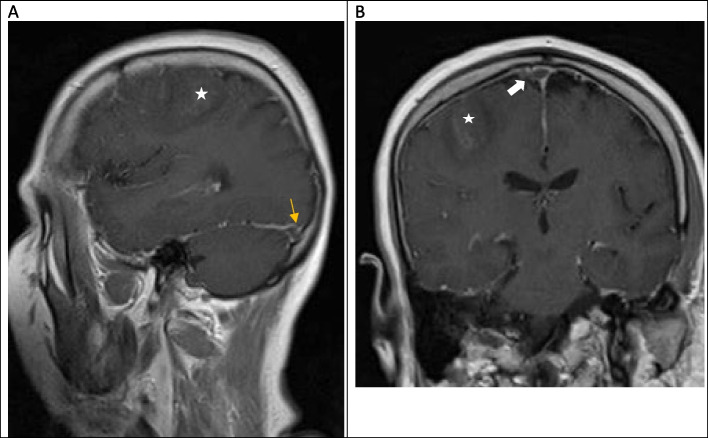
Contrast-enhanced T1-weighted MRI of the brain. A sagittal plane demonstrating a filling defect in the right transverse sinus. (Yellow arrow) and hypersignal area showing SAH (Red arrow) and surrounding hypodensity in favor of infarct (white star) B coronal plane (white arrow) showing thrombosis in the superior sagittal sinus
